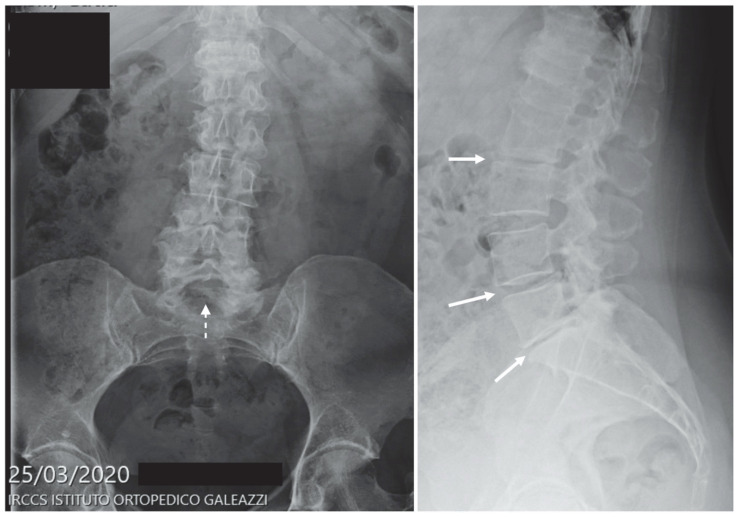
Figure shows a frontal and lateral lumbar spine X-ray examination of a patient suffering from sciatica, which presents with diffuse spondylarthrosis with lower tract spinal canal stenosis, mild scoliosis and loss of intervertebral disc space at multiple levels (arrows). Spina bifida occulta is also suspected at L5 (dashed arrow). The presence of vertebral fracture was excluded by follow-up MRI examination (not shown).
